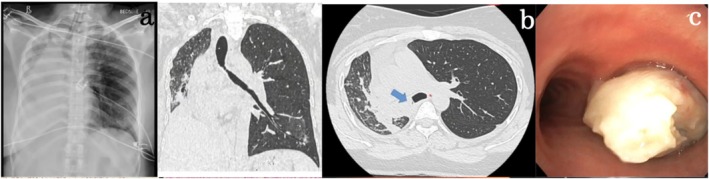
Chest Radiograph showing complete opacification of the right hemithorax (a). The lesion measuring approx. 2.3 cm × 1.3 cm in maximum dimensions with cephalon‐caudal extent of 3.2 cm in the right main bronchus. Superiorly the lesion is seen to involve upto the carina. Inferiorly the lesion is seen to extend into bronchus intermedius causing collapse of right middle, lower lobes (b). Smooth, vascular and friable growth was seen at the opening of the right mainstem bronchus; growth was seen to encroach into all the major segments of the right upper, middle and lower lobes (c).
